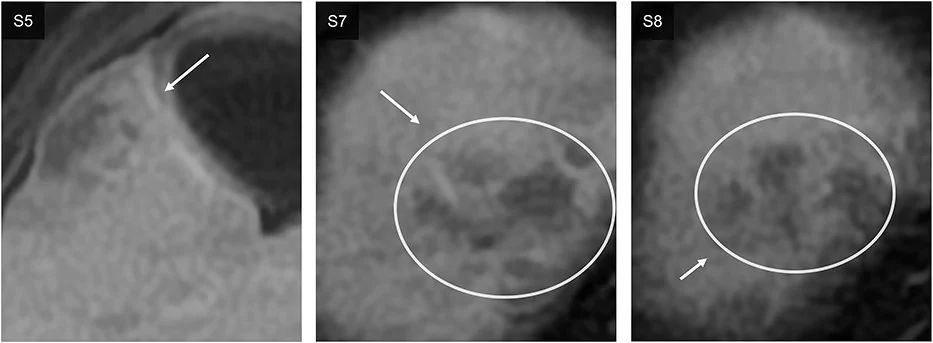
EOB-MRI at 3 months after conventional transarterial chemoembolization, in the hepatobiliary phase. The treated segment 5 lesion and non-target lesions in segments 7 and 8 remain visible.
